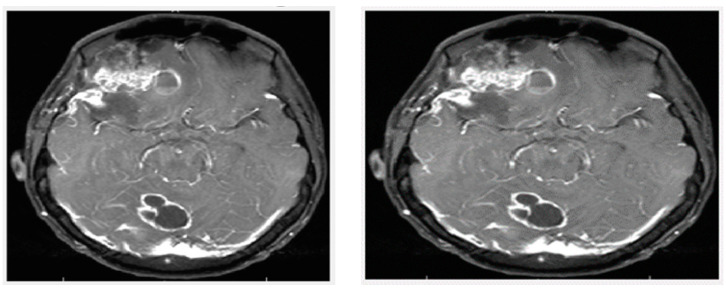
MRI image after preprocessing: (Left) Original image and (Right) Enhanced image.
